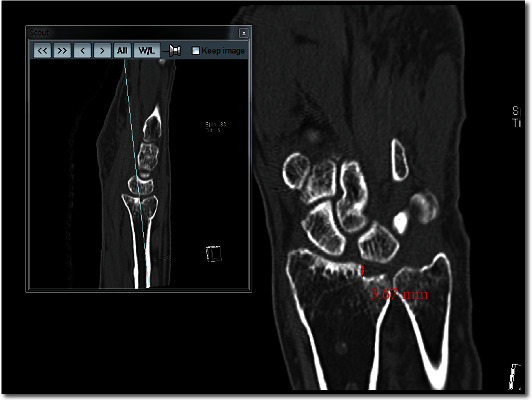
Final CT scans. Degrees of the articular gap of step-off of each involved fragment were measured.
